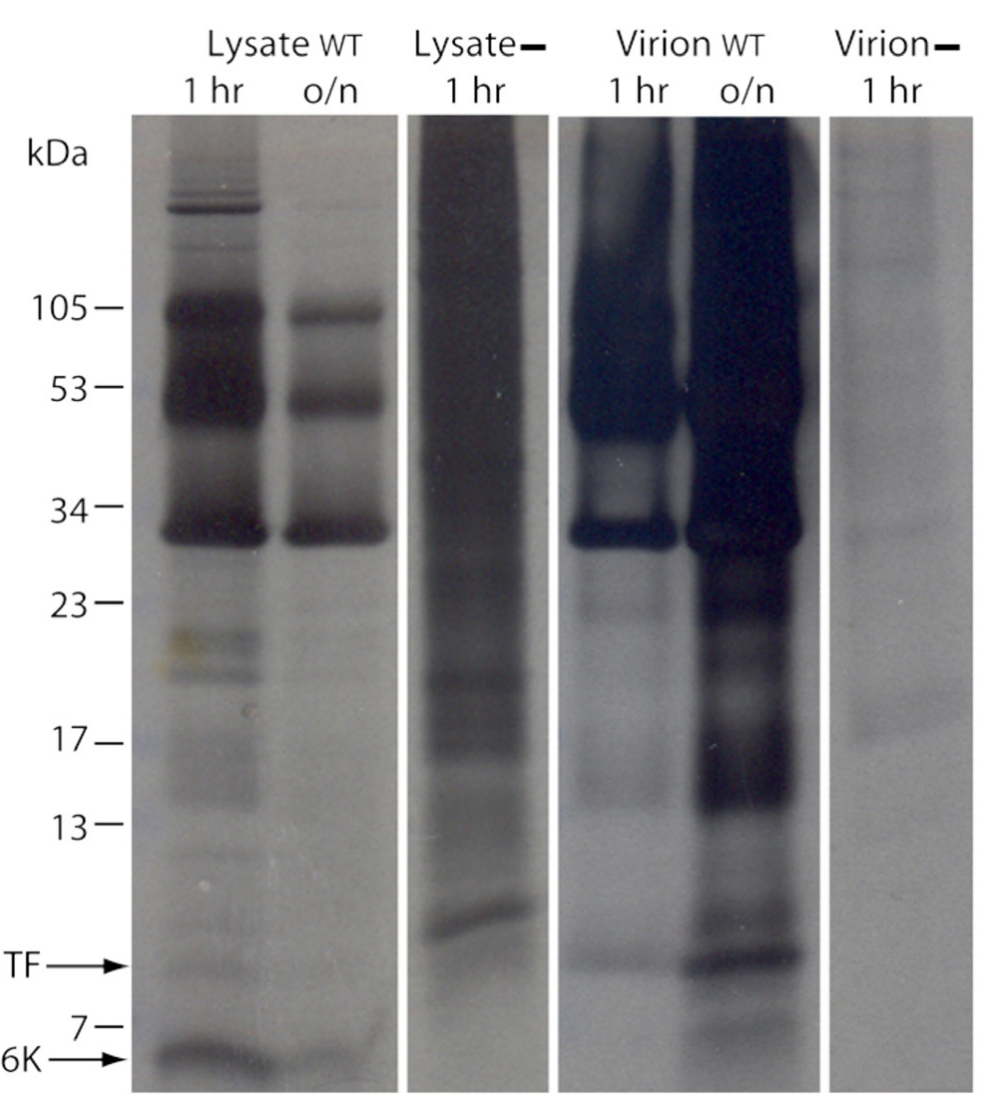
Virus-specific detection of SFV 6K and TF proteins. Lanes 1–3: total lysate from SFV-infected (WT; 1 hr and overnight, o/n) and non-infected (-) cells. Lanes 4–6: virions purified from the media (WT) and mock purified virions from non-infected cells (-). Equal amounts of transfecting RNA and cells were used for each sample. All lanes are from the same gel – exposed on x-ray film for 2 weeks to enhance the faint bands corresponding to any low molecular mass products.
